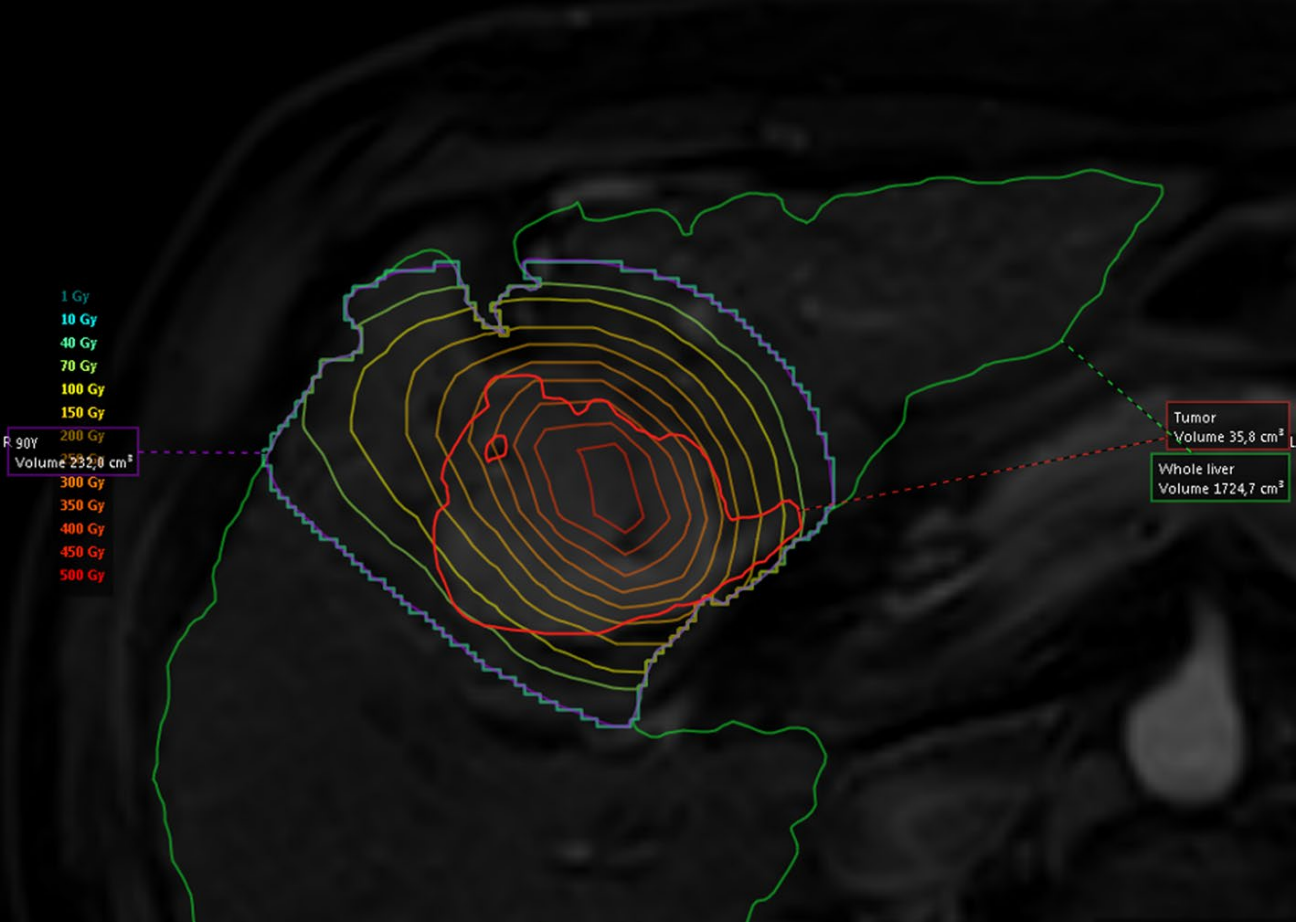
Isodose curves from ^90^Y BECT on MRI images (T2 sequence) in a 61-year-old male patient suffering from an HCC well perfused but non-homogeneously with undertreated parts (under 205 Gy)
